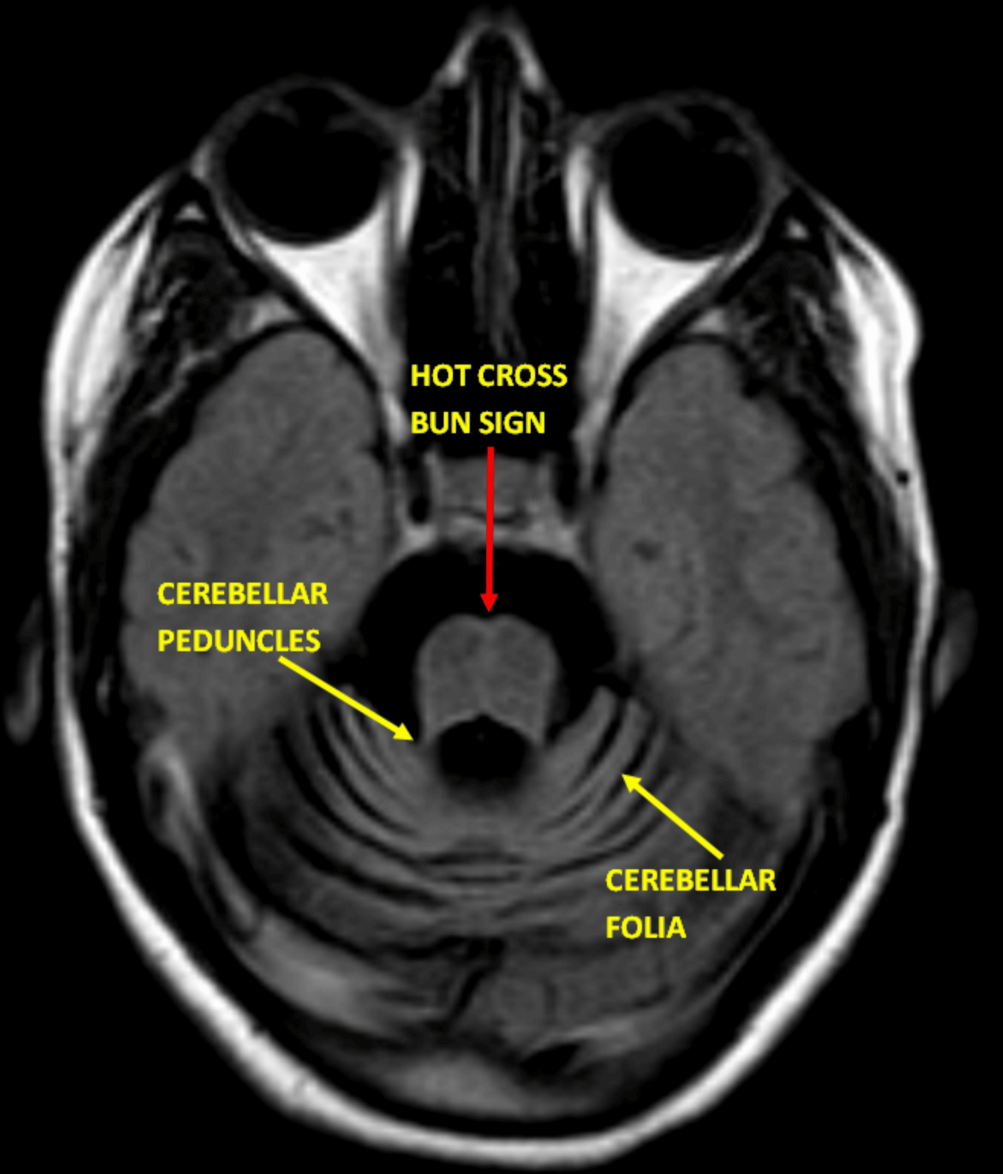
Axial FLAIR image shows atrophy involving the brainstem, cerebellum, and superior and middle cerebellar peduncles (yellow arrow). The cerebellar atrophy is evident with prominent cerebellar fissures and an enlarged fourth ventricle. Notably, the "hot cross bun" sign (red arrow) is apparent in this section across the pons, characterized by a cruciform hyperintensity that represents the degeneration of transverse pontocerebellar fibers and the pontine nuclei, a hallmark of olivopontocerebellar degeneration (OPCD). Additionally, there is a straightening of the pontomedullary angle, which is a further indicator of the atrophic changes in the brainstem. These features are highly suggestive of OPCD, reflecting the progressive neurodegenerative changes associated with this condition. The hyperintensities observed in the pons on this FLAIR image are indicative of gliosis, consistent with chronic neuronal loss. FLAIR: fluid-attenuated inversion recovery
